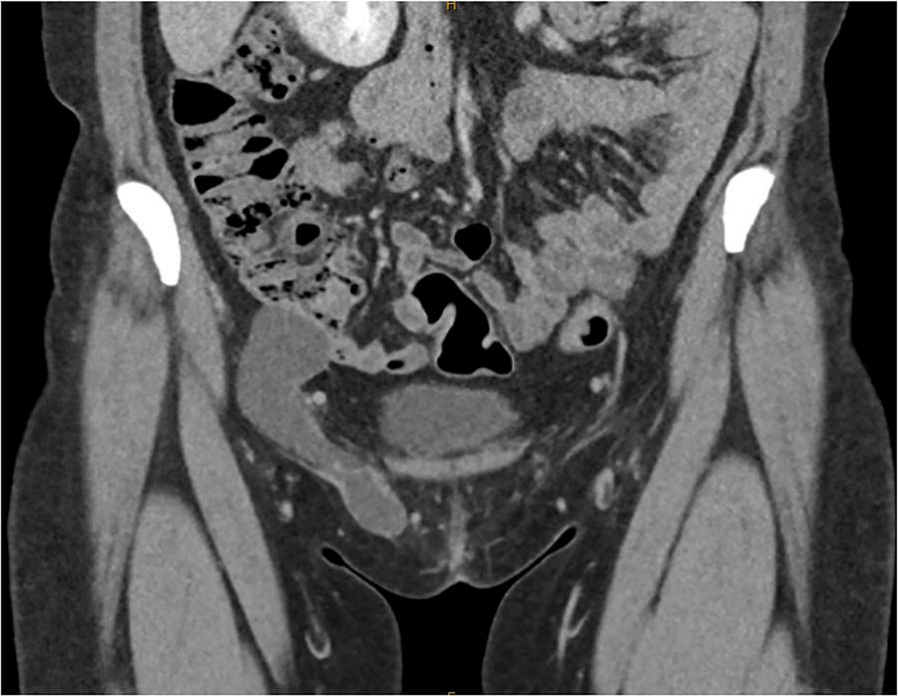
Coronal slice of CT demonstrating the bilobar right sided hydrocele of the canal of Nuck.
